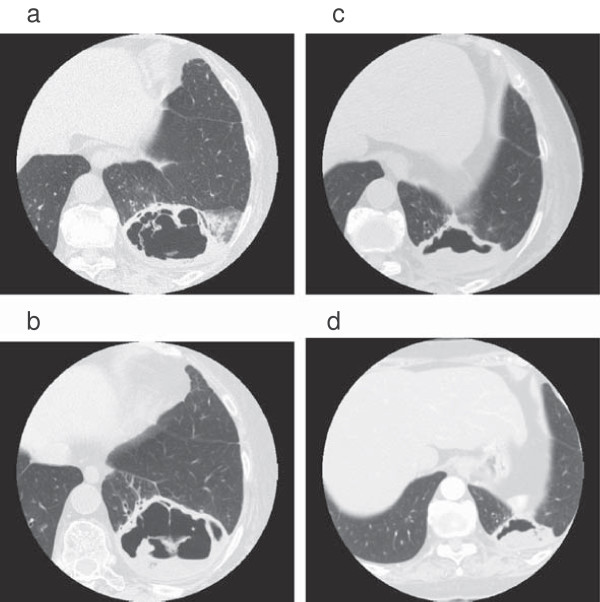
Computed tomographic images of the lung abscess on the second (a), 31^st ^(b), 79^th ^(c), and 98^th ^(d) days after admission. The maximum diameters of the lesion were 60, 87, 60, and 50mm, respectively. As shown here, the lesion was largest around the 30^th^ day after admission.
